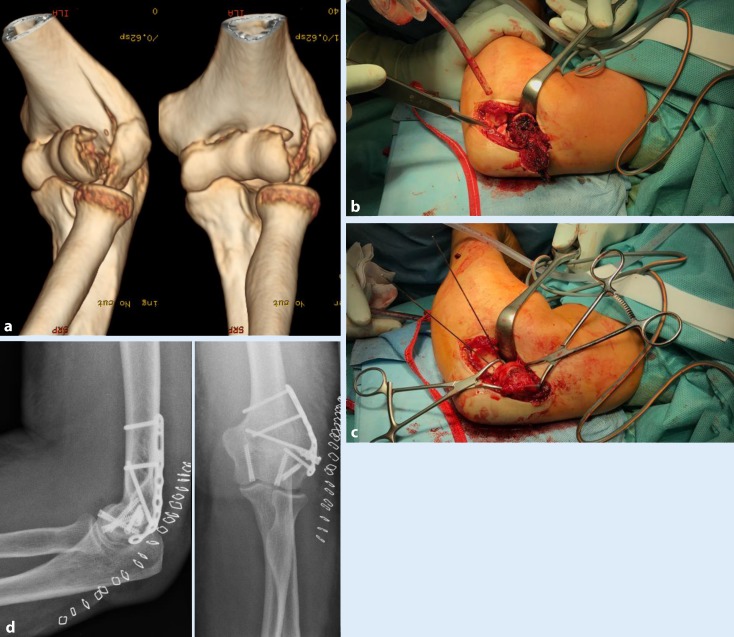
Coronal shear fracture with radial metaphyseal fracture extension, AO 13 B3/Dubberley 2B (54-year-old female patient). a Preoperative computed tomography image with three-dimensional reconstruction; b extended radial approach with surgical radial dislocation; c reduction and temporary fixation with K‑wires; d headless compression screws in anterior–posterior direction and an additive locking plate
